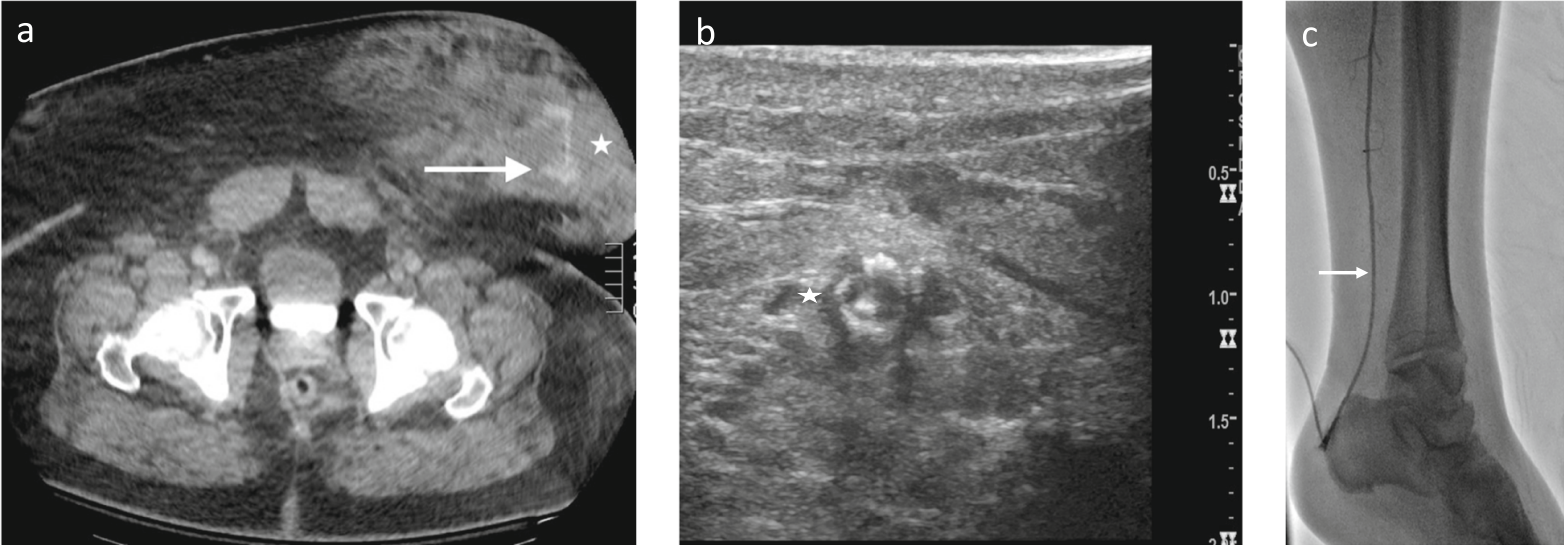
Patient 2 Seat-belt injury and ipsilateral tibial access: Axial contrast enhanced CT following seat-belt injury demonstrating contrast extravasation (arrow) in the left anterior abdominal hematoma (*) (a). Ultrasound guided access into the distal PTA (*) (b). Hand injection through vascular sheath following PTA access (c)
